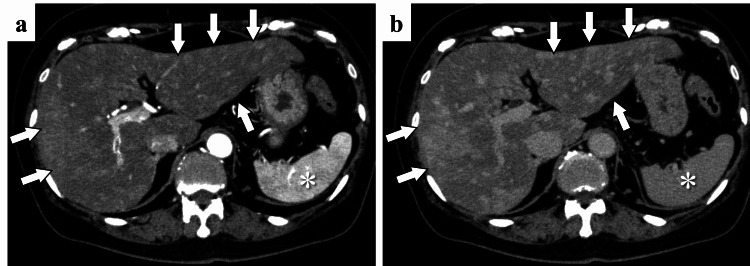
Abdominal enhanced CT performed 18 months prior to the visit to our department (a, b) Early-phase and late-phase of abdominal enhanced CT showing diffusely hypervascular liver metastases (arrows), while there was no evidence of cirrhosis or splenomegaly (*).
